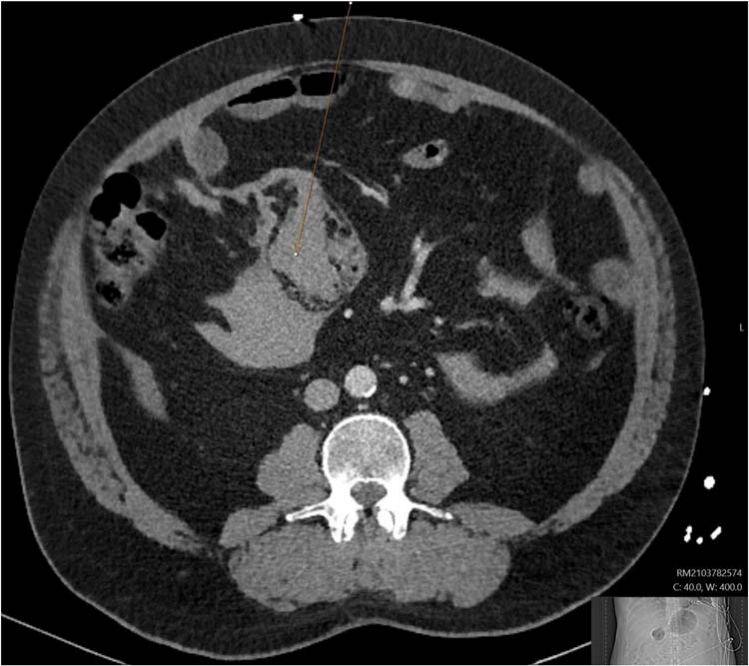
CT angiogram showing a large acute mesenteric haematoma. Figure 1 is taken from an axial section of the patient’s initial CT angiogram of the abdomen and pelvis. The arrow shown highlights an area of high-density fluid representing a 104 x 62 mm acute mesenteric haematoma.
